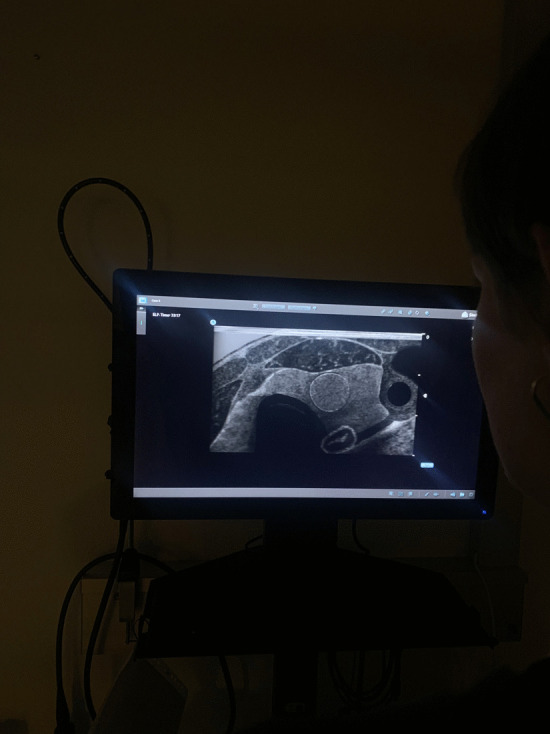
Picture of Case 5 in the neck ultrasound module of the U/S mentor. Measurements of the nodule were 0.99 cm in both length and width
